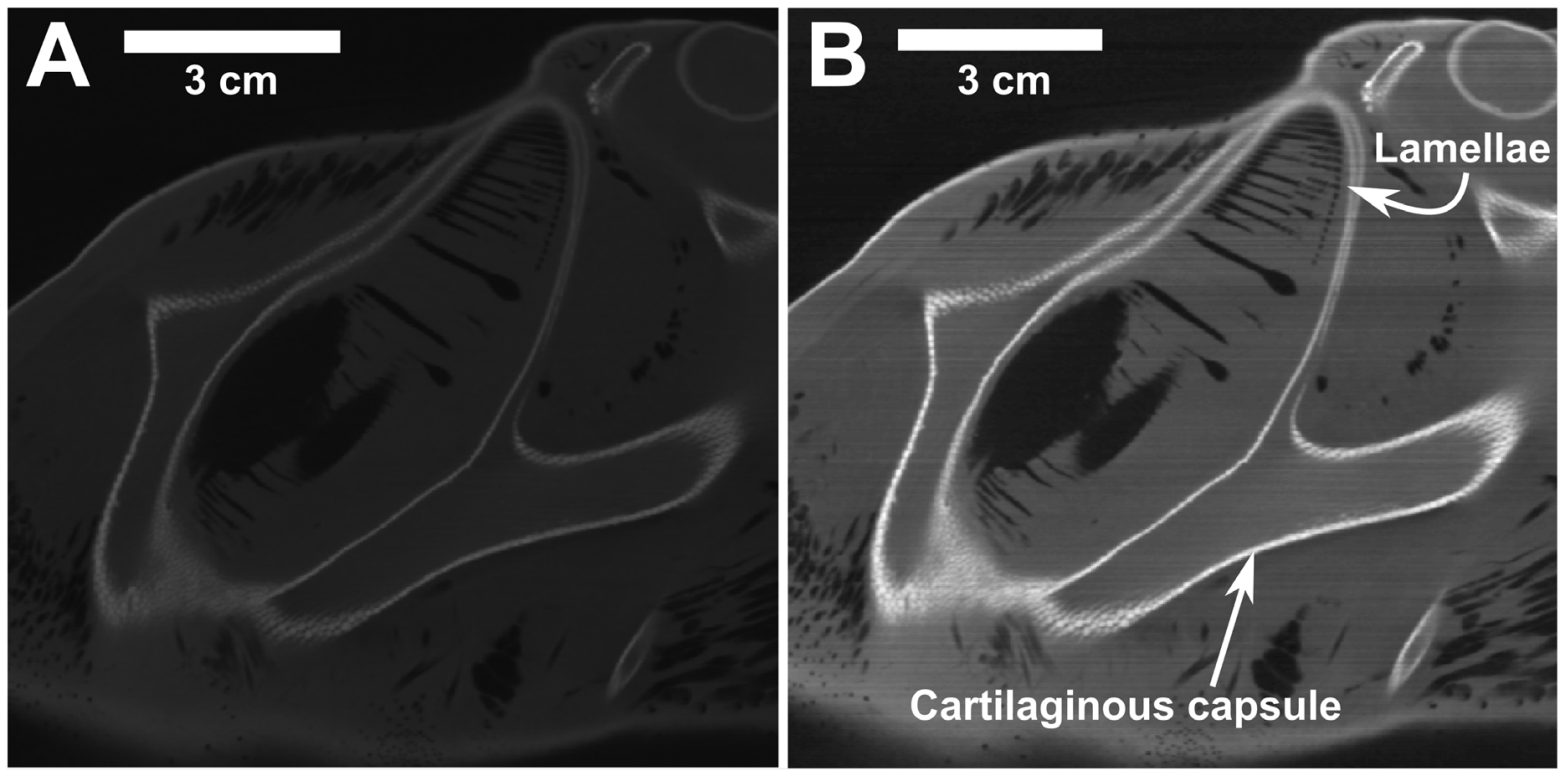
Effect of image processing on the raw CT data. Comparison of a (A) raw and (B) processed CT slice.
